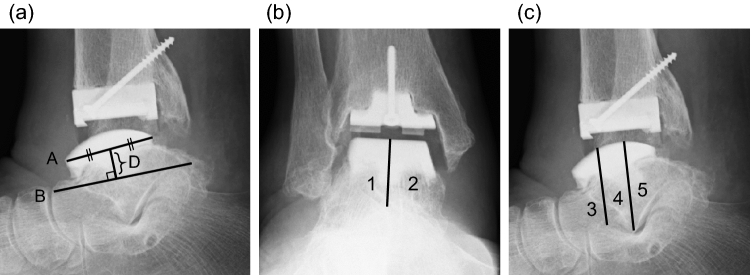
a Measurement of talar component subsidence using a sagittal plane radiograph. Line A was matched up with the talar component axis. Line B connected the dorsal aspect of the talar head and the tip of the posterior talar process. Line D was drawn perpendicular to Line B such that it meets Line A at its centre. The difference in the lengths of line D immediately after surgery and at the final follow-up was defined as talar component subsidence. b The zoning protocol for detecting locations of osteolysis. On the coronal plane radiograph, the talus was divided along the centre of the talar component, and the lateral and medial parts were assigned zones 1 and 2, respectively. c On the sagittal plane radiograph, the talar component was split into three parts vertically and the anterior, middle, and posterior regions were assigned zones 3, 4, and 5, respectively
